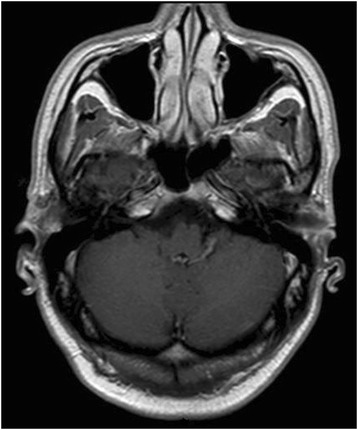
MRI of the central nervous system 3 months after amoxicillin treatment and posaconazole initiation. The imaging shows total regression of the cerebellar lesion
